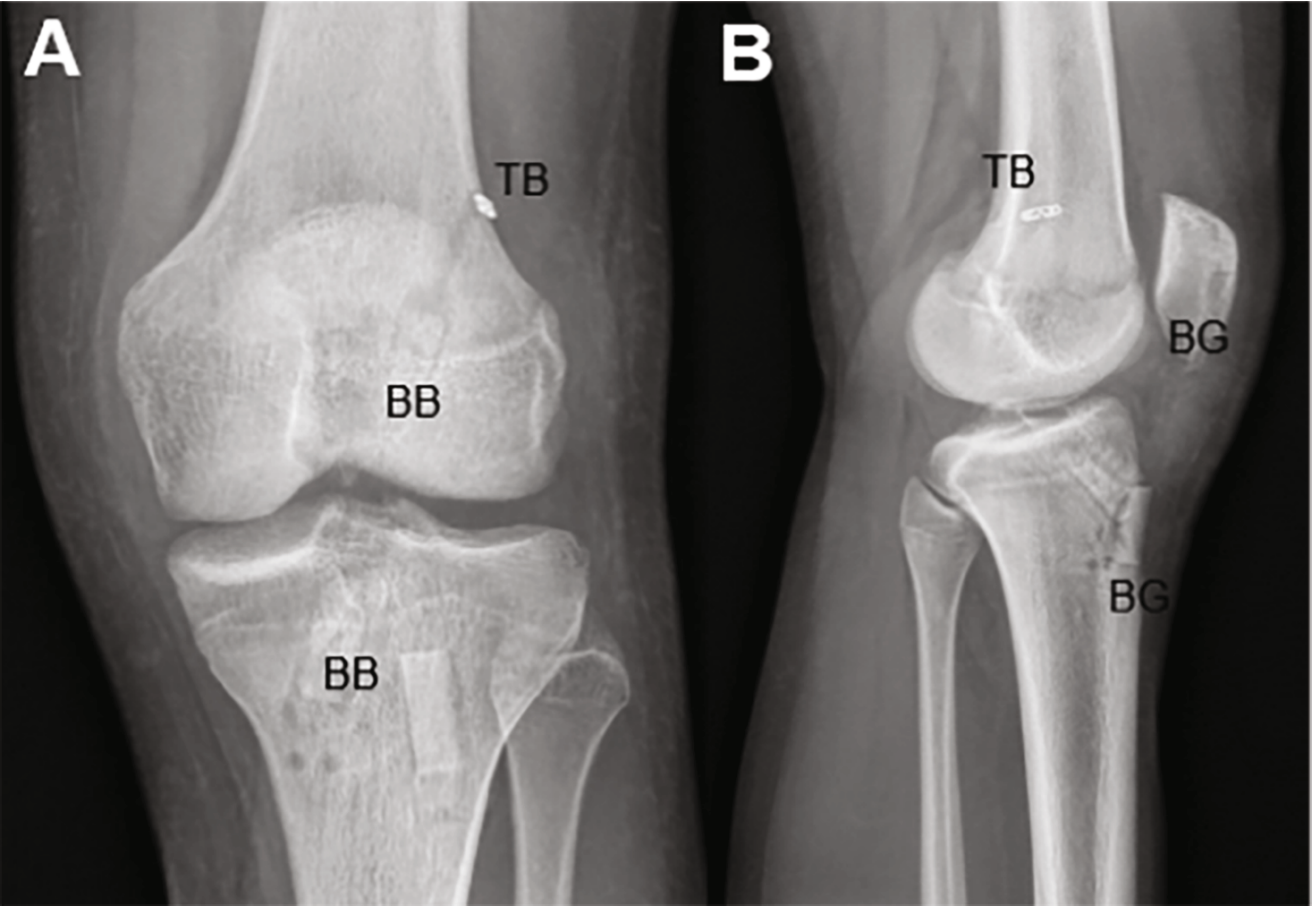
Left knee radiographs taken 2 weeks following BTB autograft ACL reconstruction with suture tape augmentation and bilateral transtibial root repairs. (A) AP radiograph of the patient's left knee demonstrating good bone block placement in the femur and tibia as well as good position of suspensory fixation button placement on the outer femoral cortex. (B) Lateral radiograph of the patient's left knee demonstrating well-placed bone grafts in the tibia and patella. TB = tight rope button; BB = bone block; BG = bone graft.
